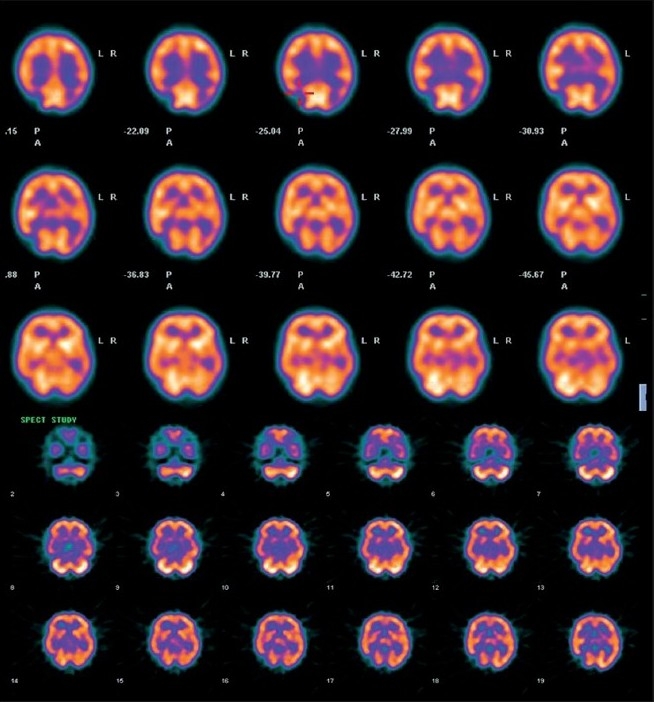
SPECT images of the brain showing hypoperfusion in the right temporo-occipital area suggestive of an old infarct, with other areas in brain parenchyma showing normal perfusion.
